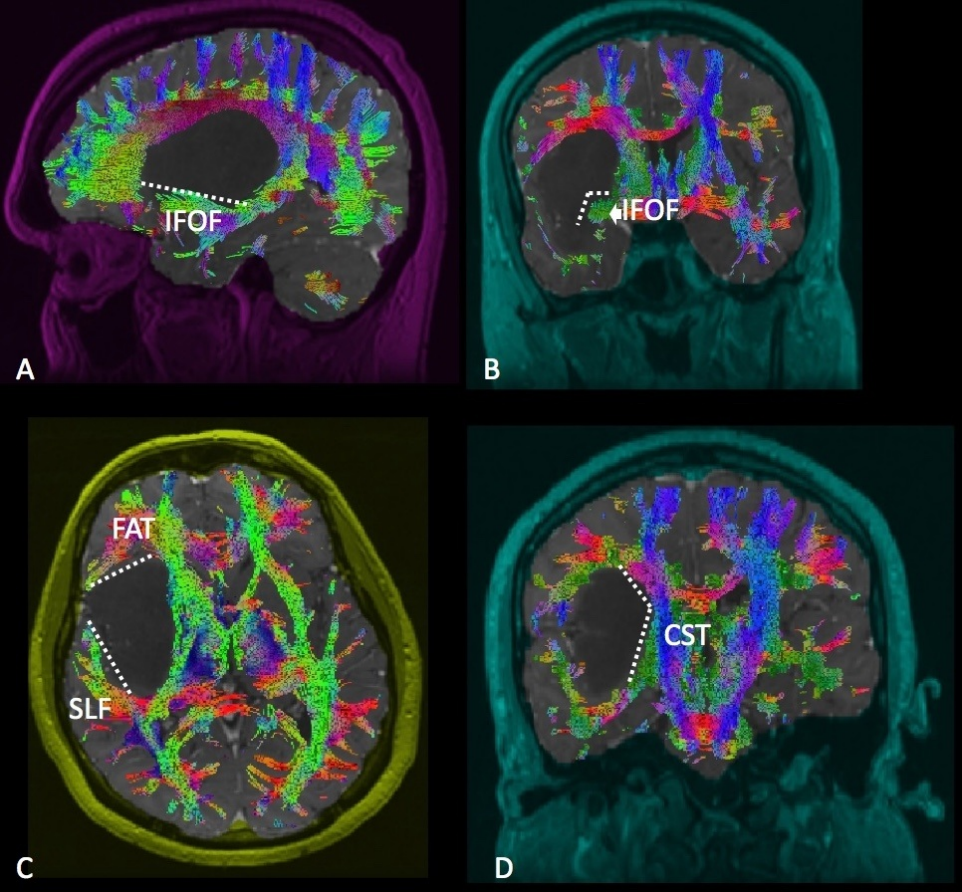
Insula Diffusion tractography enhanced T1 magnetic resonance imaging (MRI) with contrast with (A) sagittal, (B) coronal, (C) axial, and (D) coronal views demonstrating an insular glioma in relation to the surrounding white matter tracts. Labeled white matter tracts associated are shown as follows: superior longitudinal fasciculus (SLF), corticospinal tract (CST), inferior fronto-occipital tract (IFOF), and frontal aslant tract (FAT). The disconnection pattern is shown as a white dotted line.
